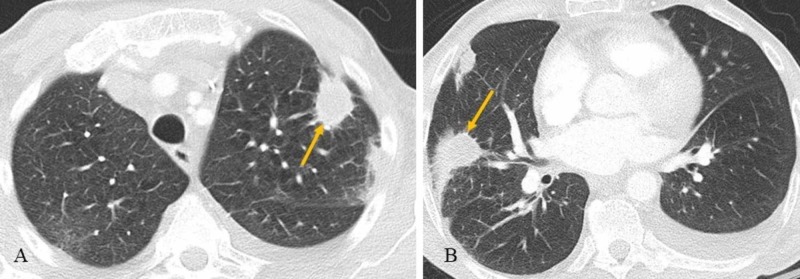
Axial (A and B) chest CT Multifocal bilateral airspace disease with areas of interval cavitation (arrows), consistent with evolving and partially treated sequela of multifocal infection. There are also small bilateral pleural effusions, greater on the left.
